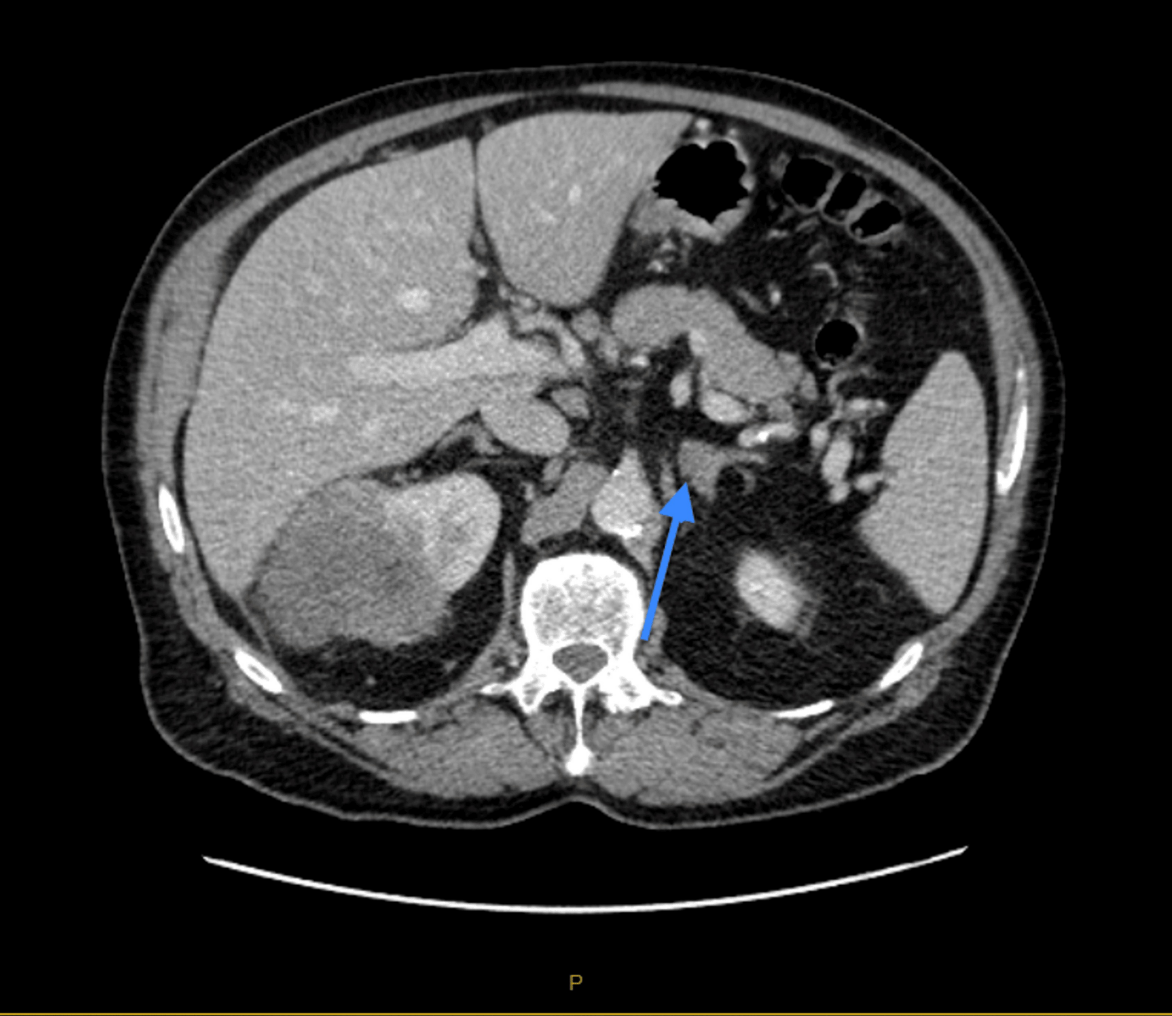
CT abdomen and pelvis with intravenous contrast demonstrating 15 mm nodule on the left adrenal gland (arrow).
